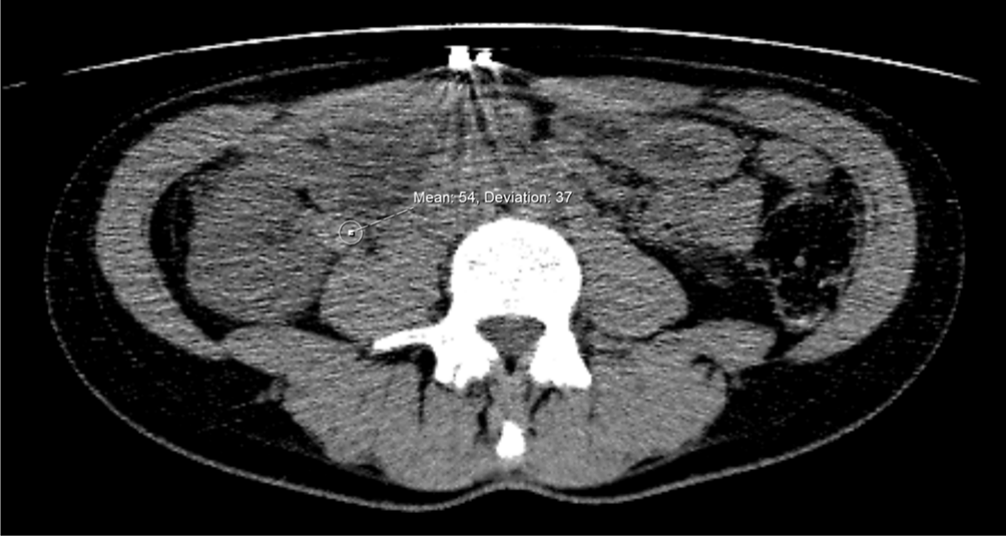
CT-KUB demonstrating a 2.2 × 2.4 cm branching high attenuation opacity
